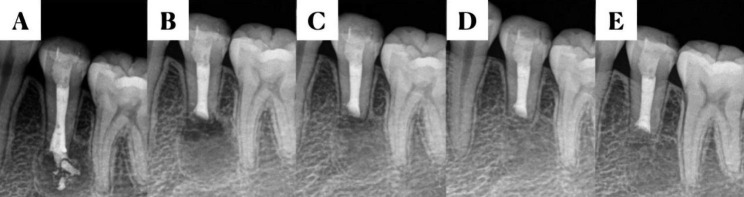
Changes in the periapical tissue after endodontic microsurgery upon the failure of the apical barrier technique. (A) Preoperative radiograph, (B) postoperative radiograph, and (C) follow-up radiographs after 3 months, (D) 6 months, and (E) 1 year
